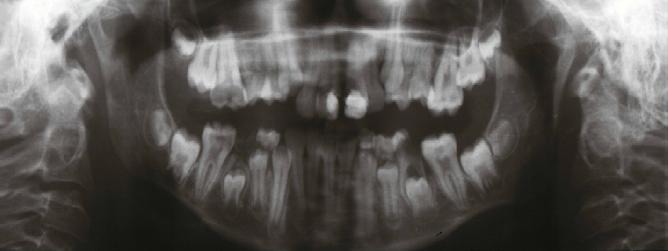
Panoramic radiograph of the patient’s dentition, demonstrating delayed dental development and deep carious lesions on left upper and lower permanent first molars and lower right second primary molar.
